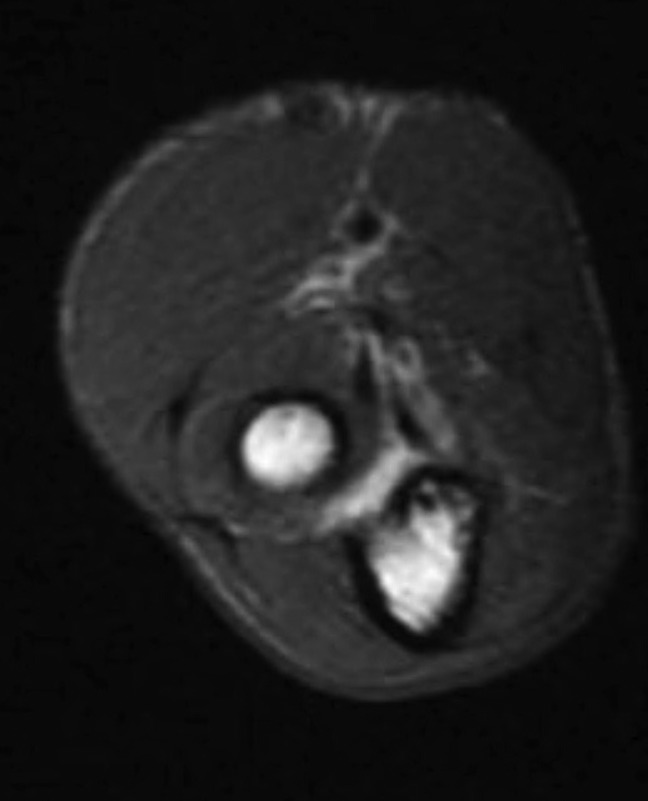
Axial T2 1.5-Tesla MRI of the right upper arm showing an intact distal biceps tendon. The image was found to be out of plane with some motion artifact, thus obscuring the injury to the short head of the biceps discovered intraoperatively.
